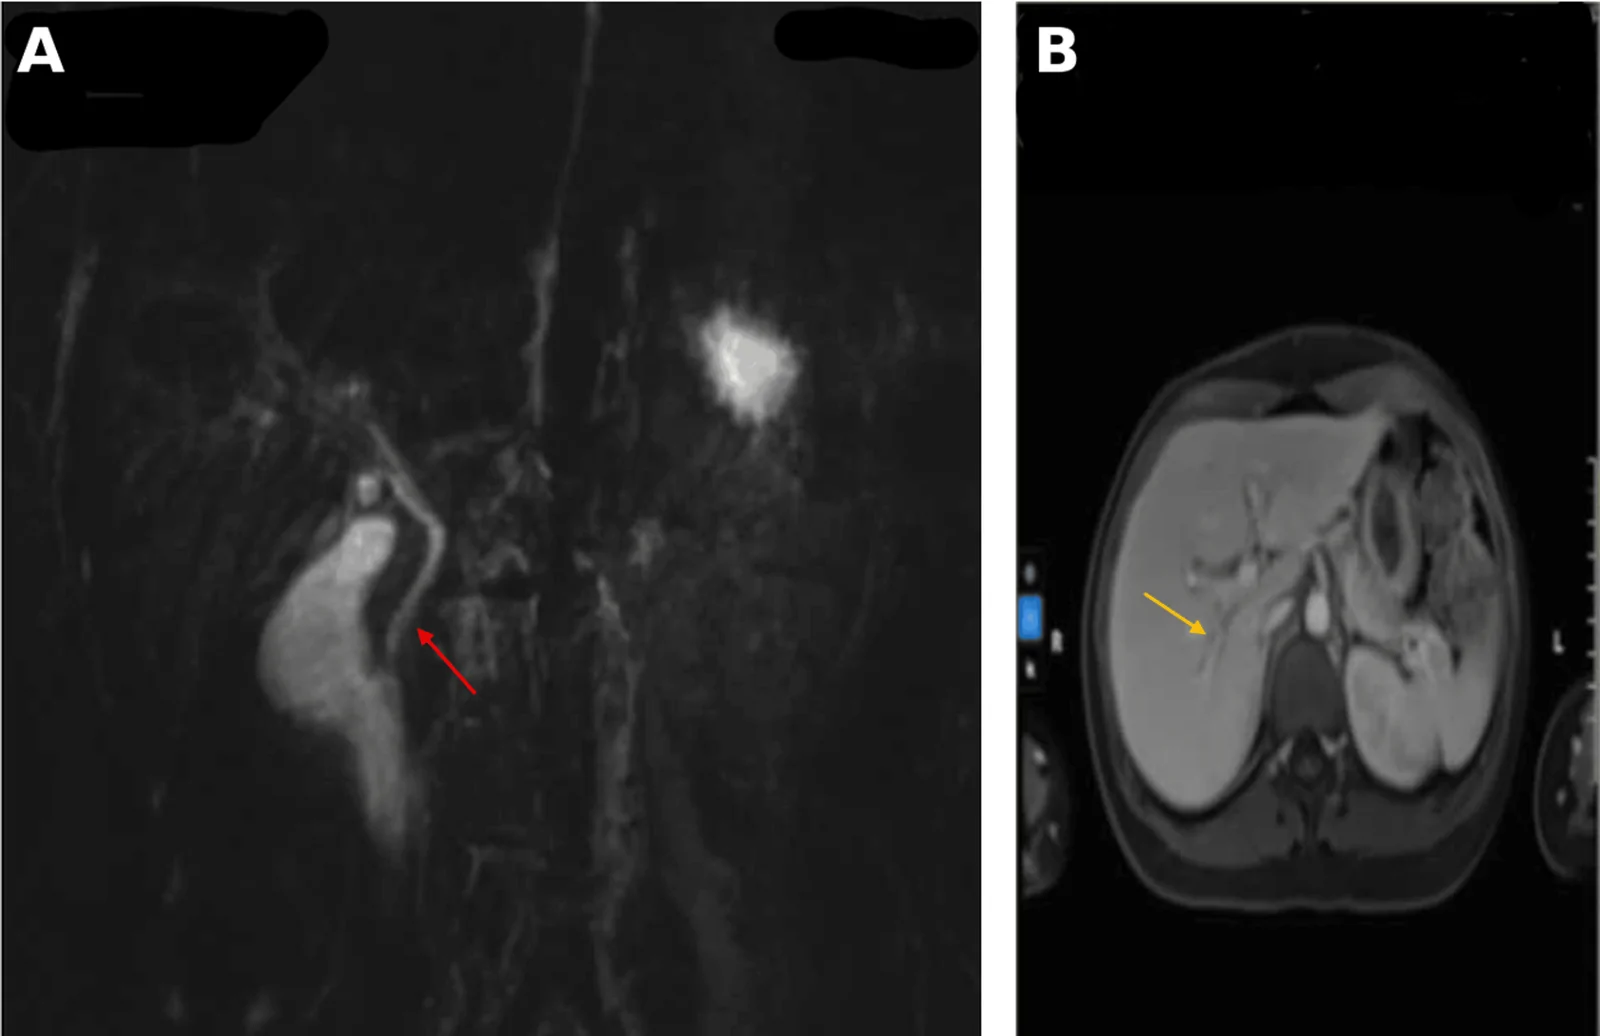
Abdominal magnetic resonance imaging findings (A) Magnetic resonance cholangiopancreatography showing a nondilated common bile duct (red arrow). (B) Axial magnetic resonance image demonstrating periportal edema (yellow arrow).
